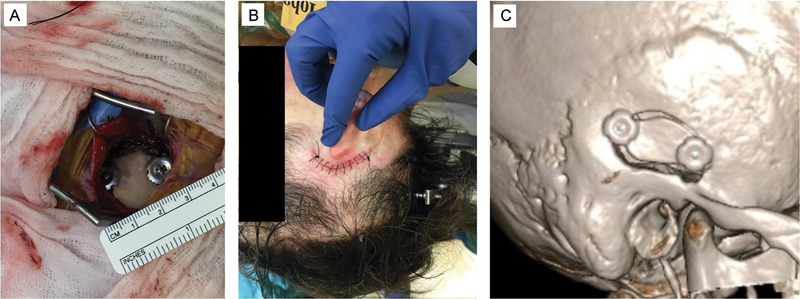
Representative views of intraoperative craniotomy (A), incision at closure (B), and postoperative three-dimensional computed tomography scan showing the right-sided craniotomy (C).
